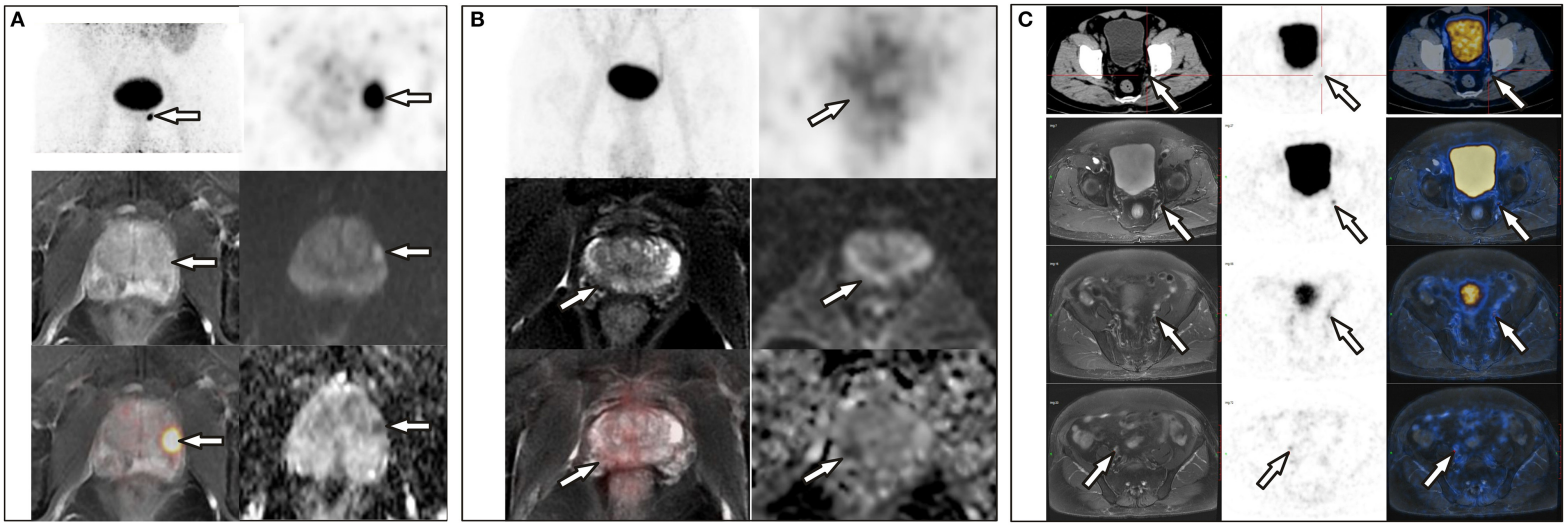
(A) A 72-year-old man with a PSA level of 21.26 ng/L underwent ^68^Ga-PSMA PET/CT and delayed pelvic PET/MR. Positive PSMA uptake was revealed in the left peripheral zone, consistent with the signal change in mpMR. (B) A 68-year-old man with proven prostate cancer in the right lobe underwent PET/MR for staging. Axial T2-weighted image shows an ill-defined hypointense lesion in the right peripheral zone with corresponding hypo-intensity on the apparent diffusion coefficient map. No significant hyperintense signal was observed on DW images (*b* = 1,000 s/mm^2^). This was assigned a PI-RADS score of 4, but negative PSMA uptake was observed with diffuse ^68^Ga-PSMA uptake in the prostate (SUVmax, 4.10). (C) A 71-year-old man with proven prostate cancer after prostate transurethral resection, ^68^Ga-PSMA PET/CT and pelvic PET/MR were performed for staging. PET/CT revealed only one small lymph node in the left pelvic cavity, which was revealed more clearly on PET/MR, and more lesions were revealed on PET/MR, which were proved to be metastases after surgery.
